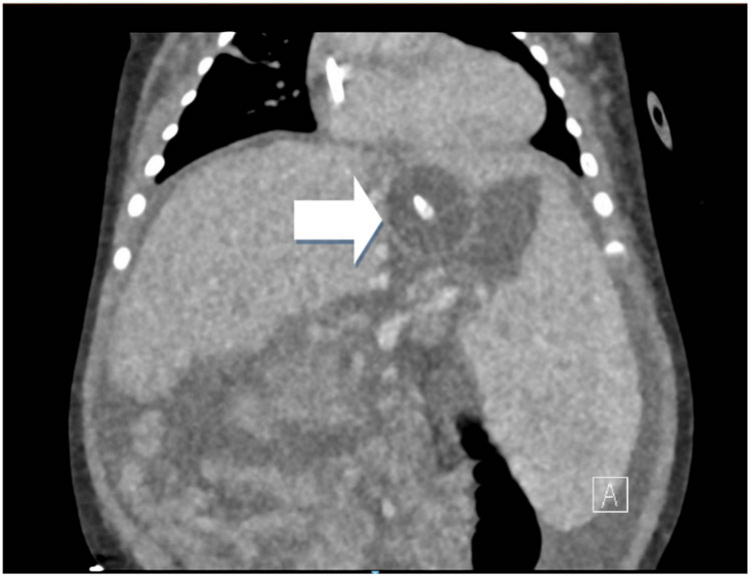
Computed tomography angiography obtained for evaluation of splenic renal shunt with visualization of foley catheter balloon inflated at the gastroesophageal junction as demonstrated by the arrow.
